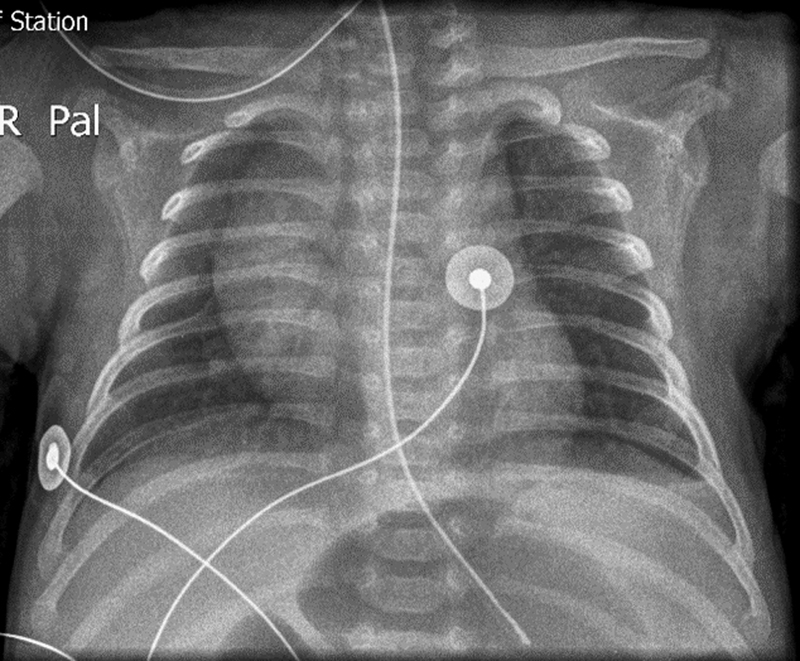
Chest X-ray at 1-year of age with no signs of diaphragmatic hernia.
